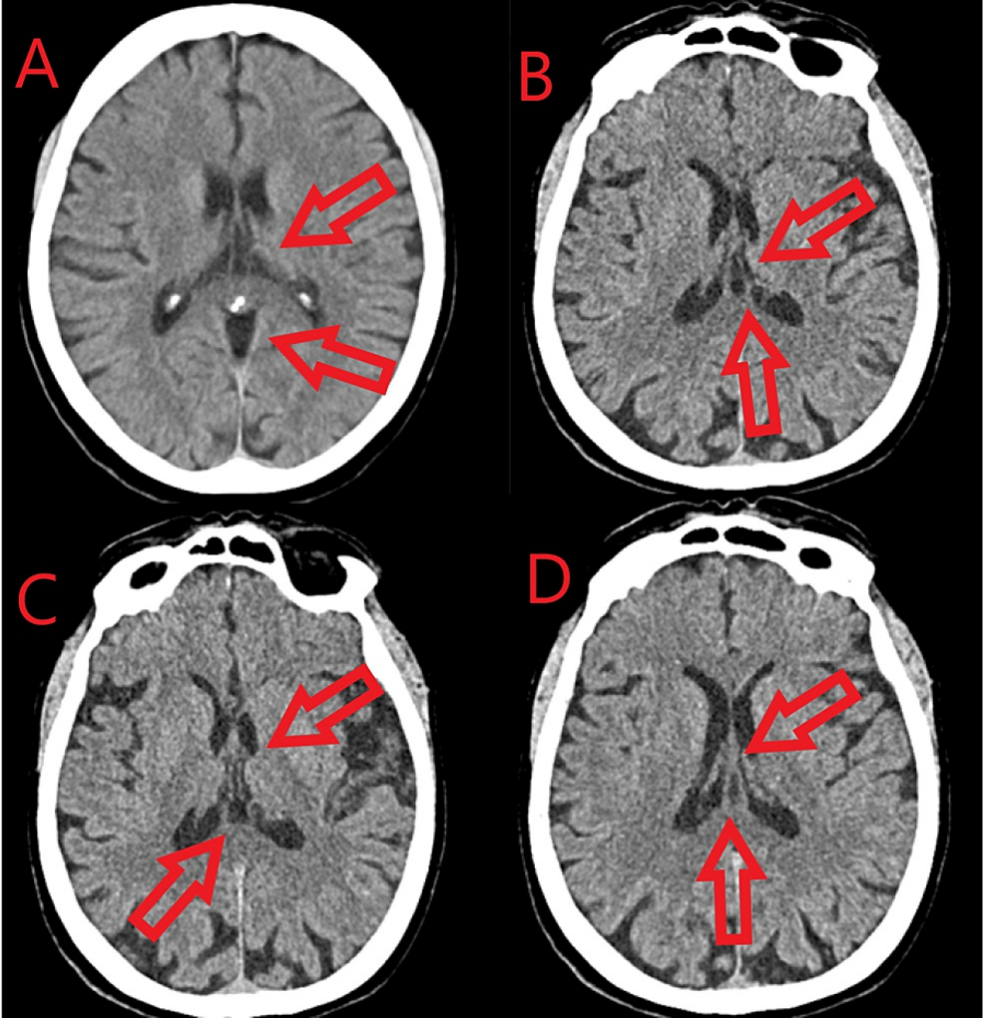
CT scan (axial plane) (A, B, C, D) Dilated subarachnoid spaces of the convexity and a CV cyst CV, cavum vergae
